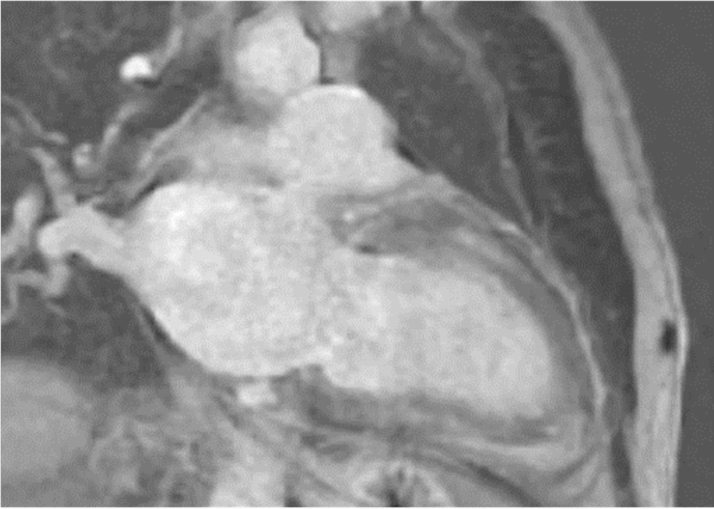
Cardiac Magnetic Resonance Imaging of Patient 1 Showing Patchy Late Gadolinium Enhancement
